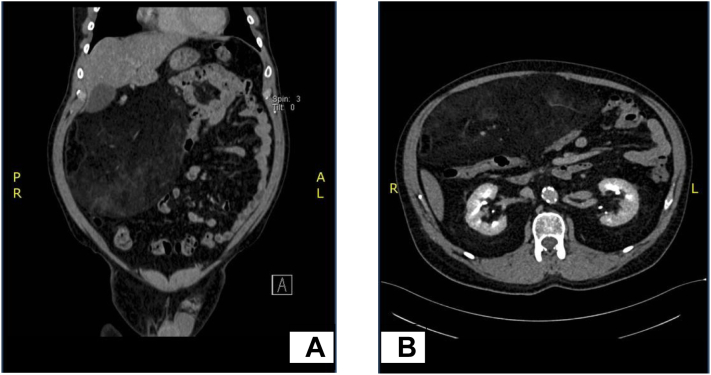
Omental lipoma case. A: Coronal abdominal CT scan demonstrating a well-defined heterogeneous lesion associated with mass effects compressing the transverse colon. B: Axial CT scan shows lobulated and encapsulated lesions.
